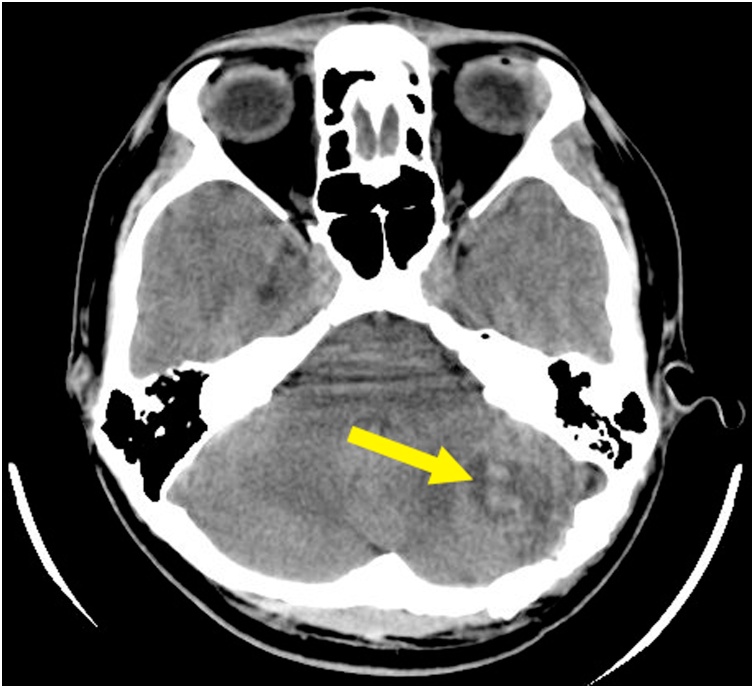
Thirty day postoperative enhanced head CT images showed a formation cystic mass in the left lobe of the cerebellum with blood density lesions and visible ring enhancement. These features suggested a formation of cerebellar abscess.
